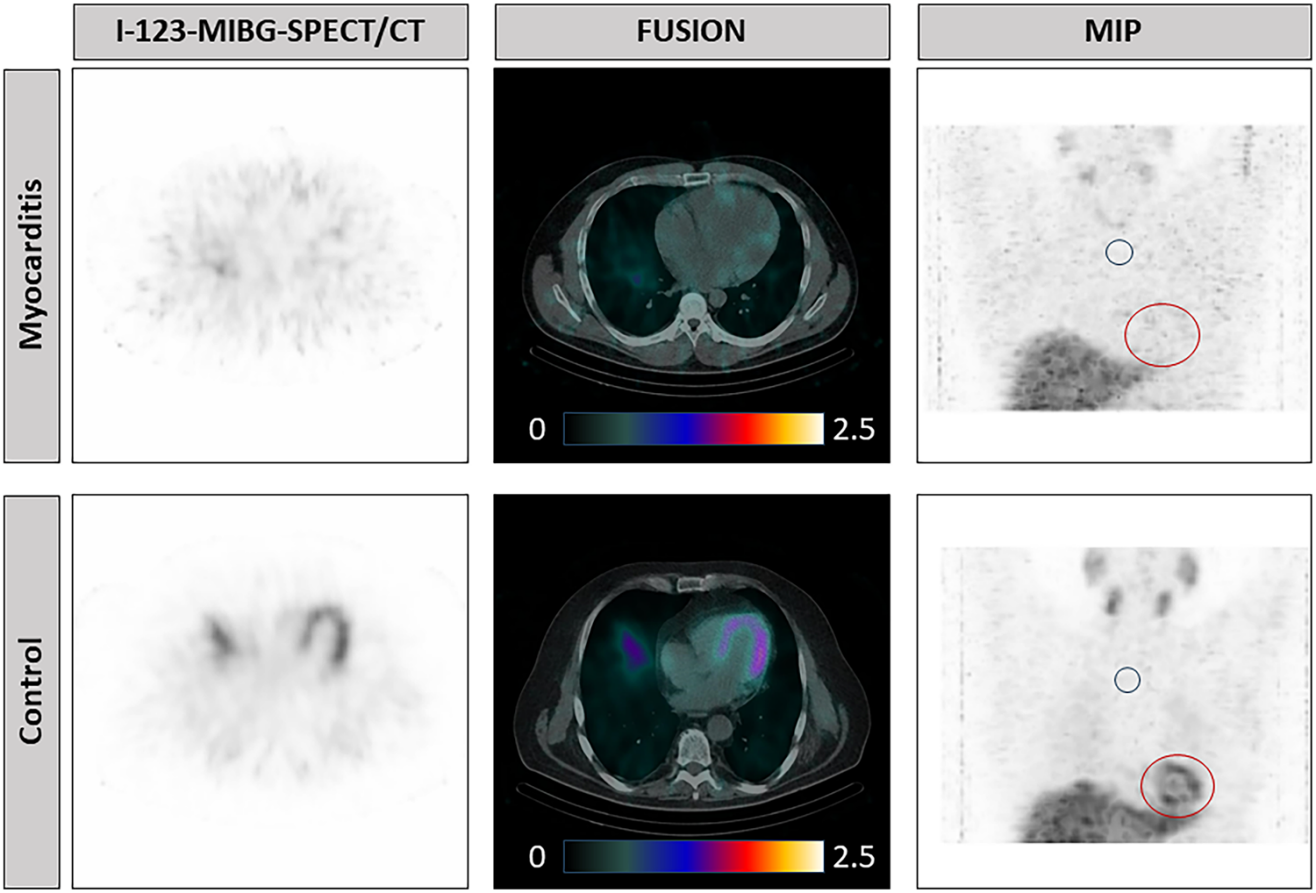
Case example of ^123^I-MIBG SPECT/CT in a patient with myocarditis and control patient 4 h p.i..MIP, maximum intensitiy projection. Color scale in KBq/ml
